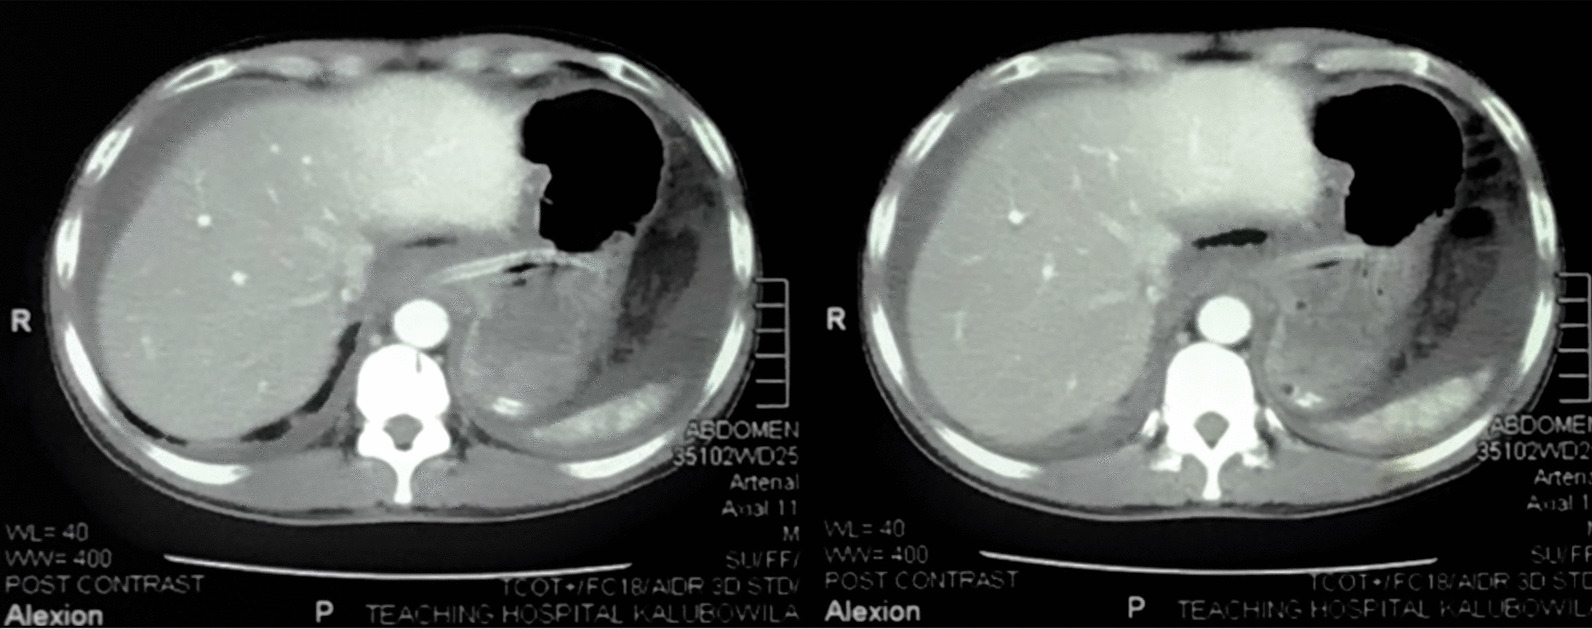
Contrast-enhanced computed tomography of the abdomen showing dilated small bowel loops
